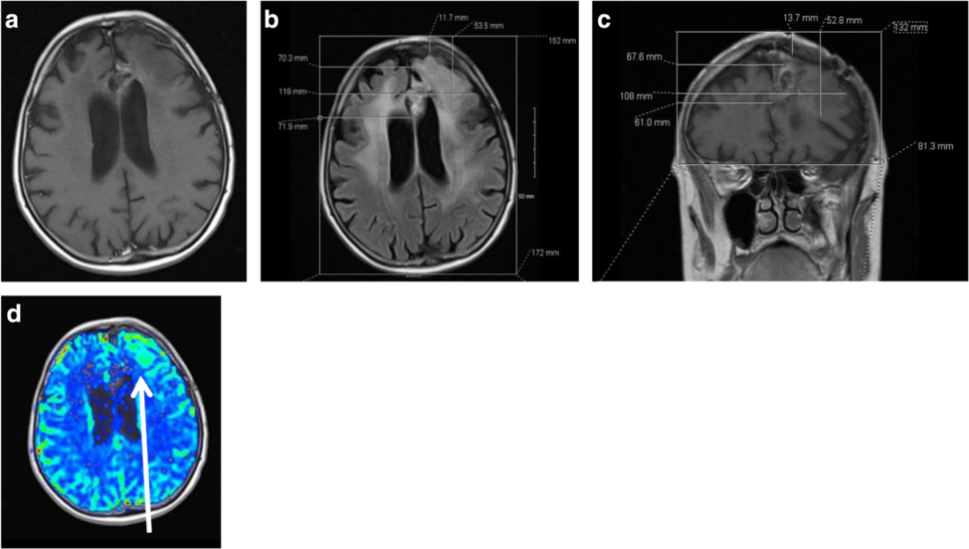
a, b, c & d. Figures a-d depict treatment planning in a recurrent glioblastoma patient presenting with an area of increased perfusion on sequential imaging. Figure a shows lack of enhancement on the axial T1 postcontrast sequence. Figure b shows axial treatment planning performed on the corresponding slice in the FLAIR sequence, where diffuse signal abnormality is present. Measurements are obtained from a reference frame drawn at the level of the scalp. Tumor coordinates are determined in a standard manner correlating the region of FLAIR signal abnormality with the area of increased perfusion observed on MR perfusion (figure d). Figure c shows the corresponding area of hypodensity on the coronal T1 with contrast sequence which is used for treatment planning in the absence of a coronal FLAIR sequence. All measurements are obtained from a reference frame drawn at the level of scalp, extending inferiorly to the lowest margin of frontal lobe. In anterior coronal sections, the reference frame should encompass all of the cerebrum at this level. Tumor location measurements are obtained in a standard fashion extending from the right frame to the edges of the T1 hypo intensity. Figure d shows an area of increased perfusion (*white arrow*) in the left frontal lobe on MR perfusion indicative of tumor recurrence
